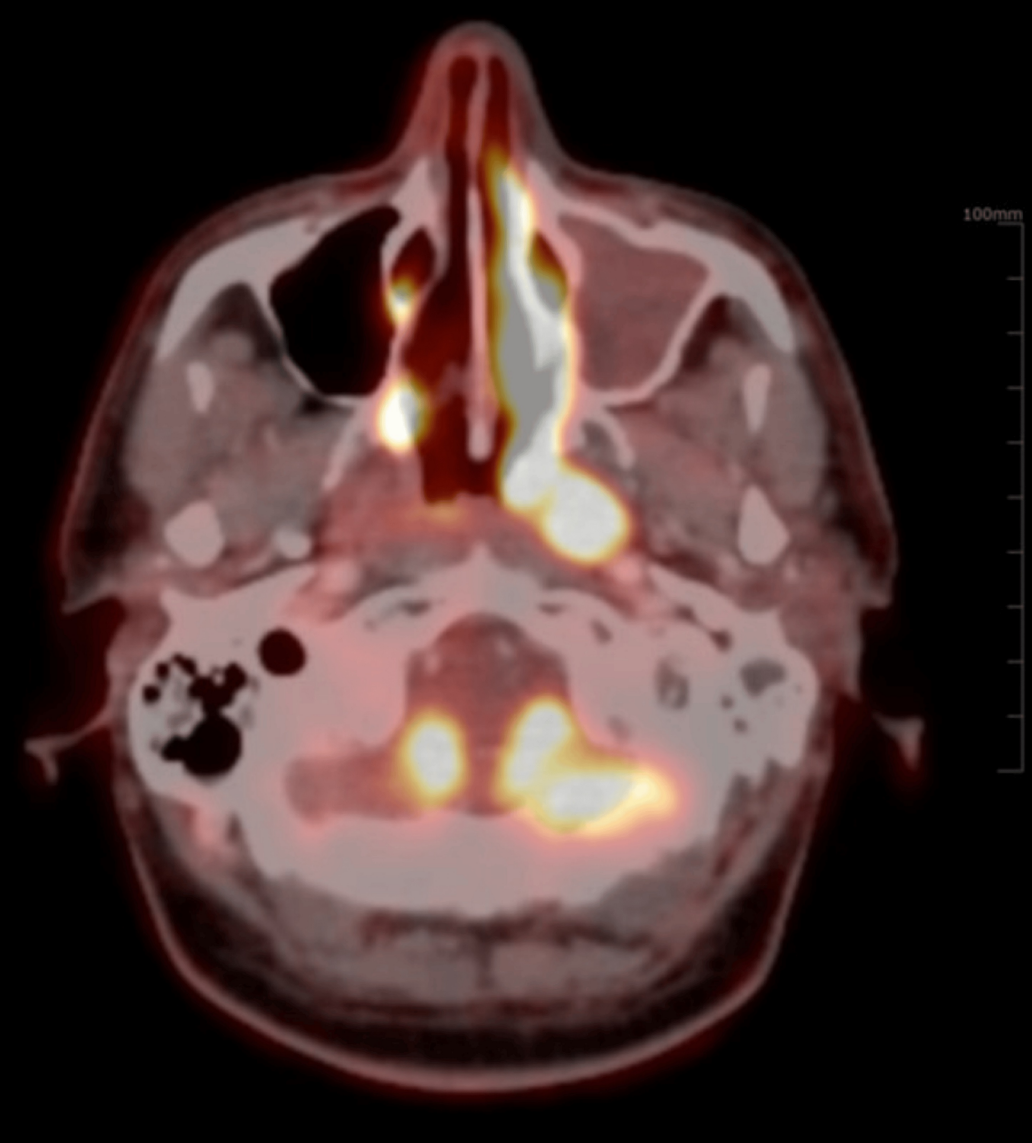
Transaxial image showing increased FDG concentration in the heterogeneously enhanced soft tissue mass in the left lateral wall of the nasopharynx, obliterating the Fossa of Rosenmuller and left Eustachian tube
